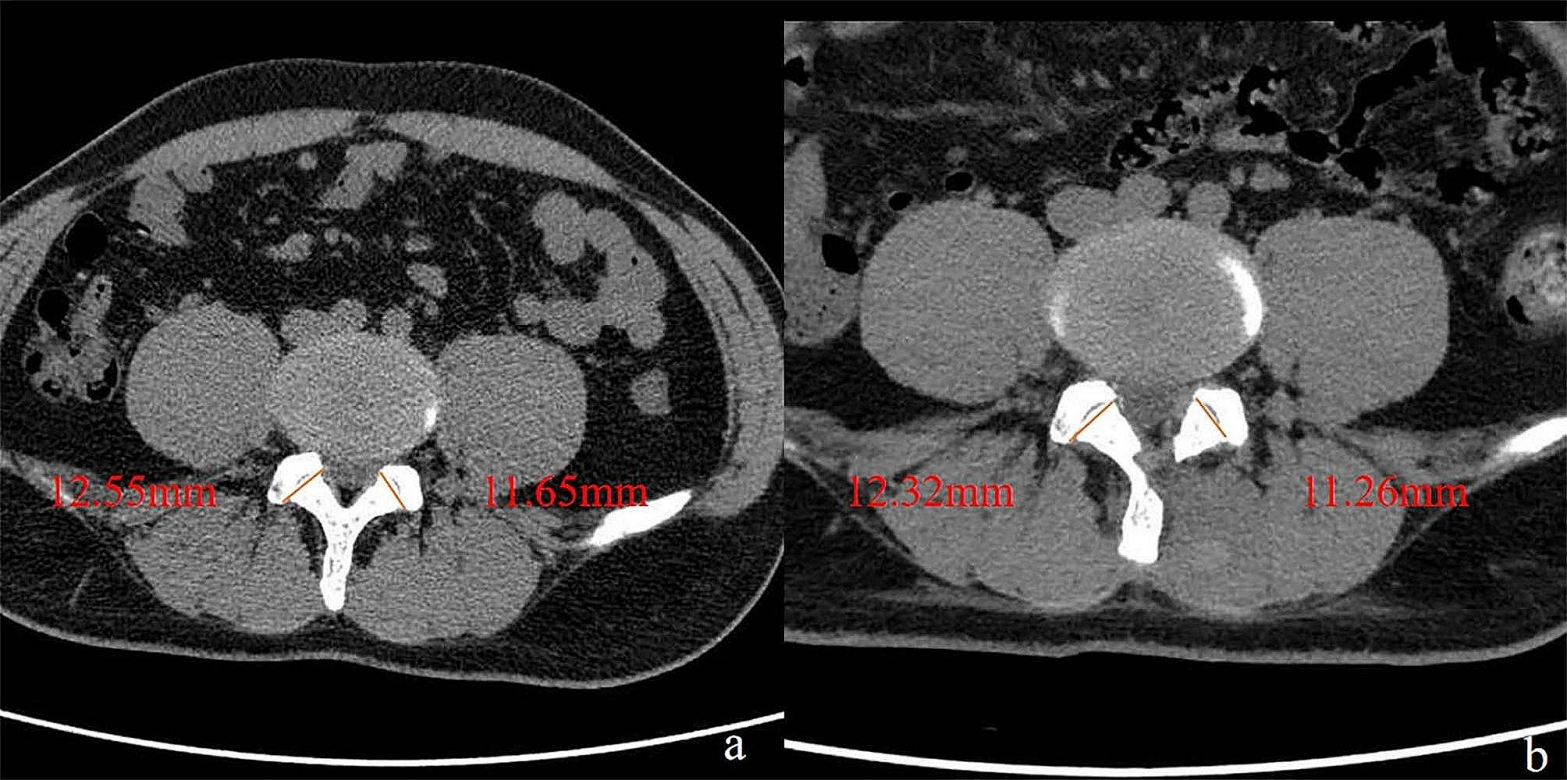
Measuring the length of facet joint plane and calculated the ratio of ipsilateral/contralateral the length of facet joint plane in preoperative (a) and postoperative axial CT images (b). Preoperative ratio is 0.93 (= 11.65 [contralateral]:12.55 [ipsilateral]). Postoperative ratio is 0.91 (= 11.26:12.32). Reduction rate is 2.15% ([0.93 − 0.91/0.93] × 100)
